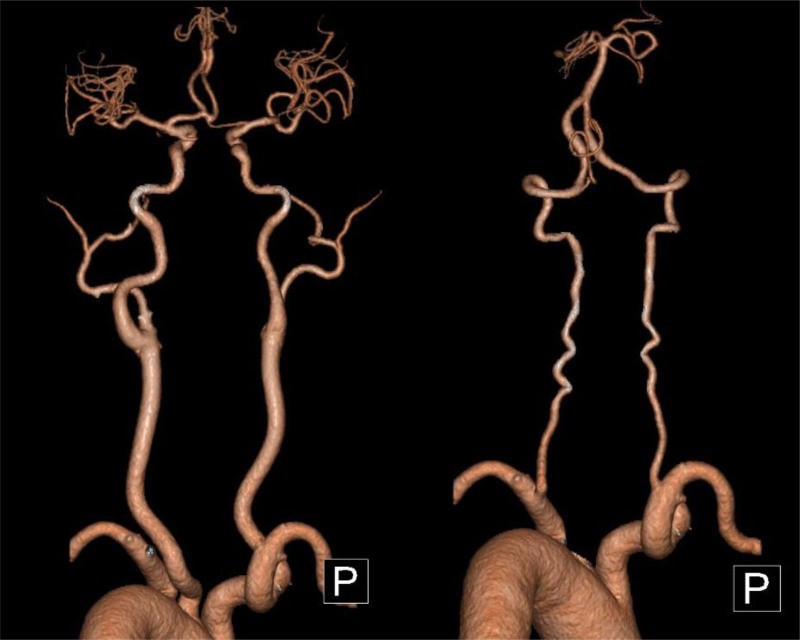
CTA of the brain and neck does not show intracranial and extracranial large artery stenosis. CTA = computed tomography angiography.
